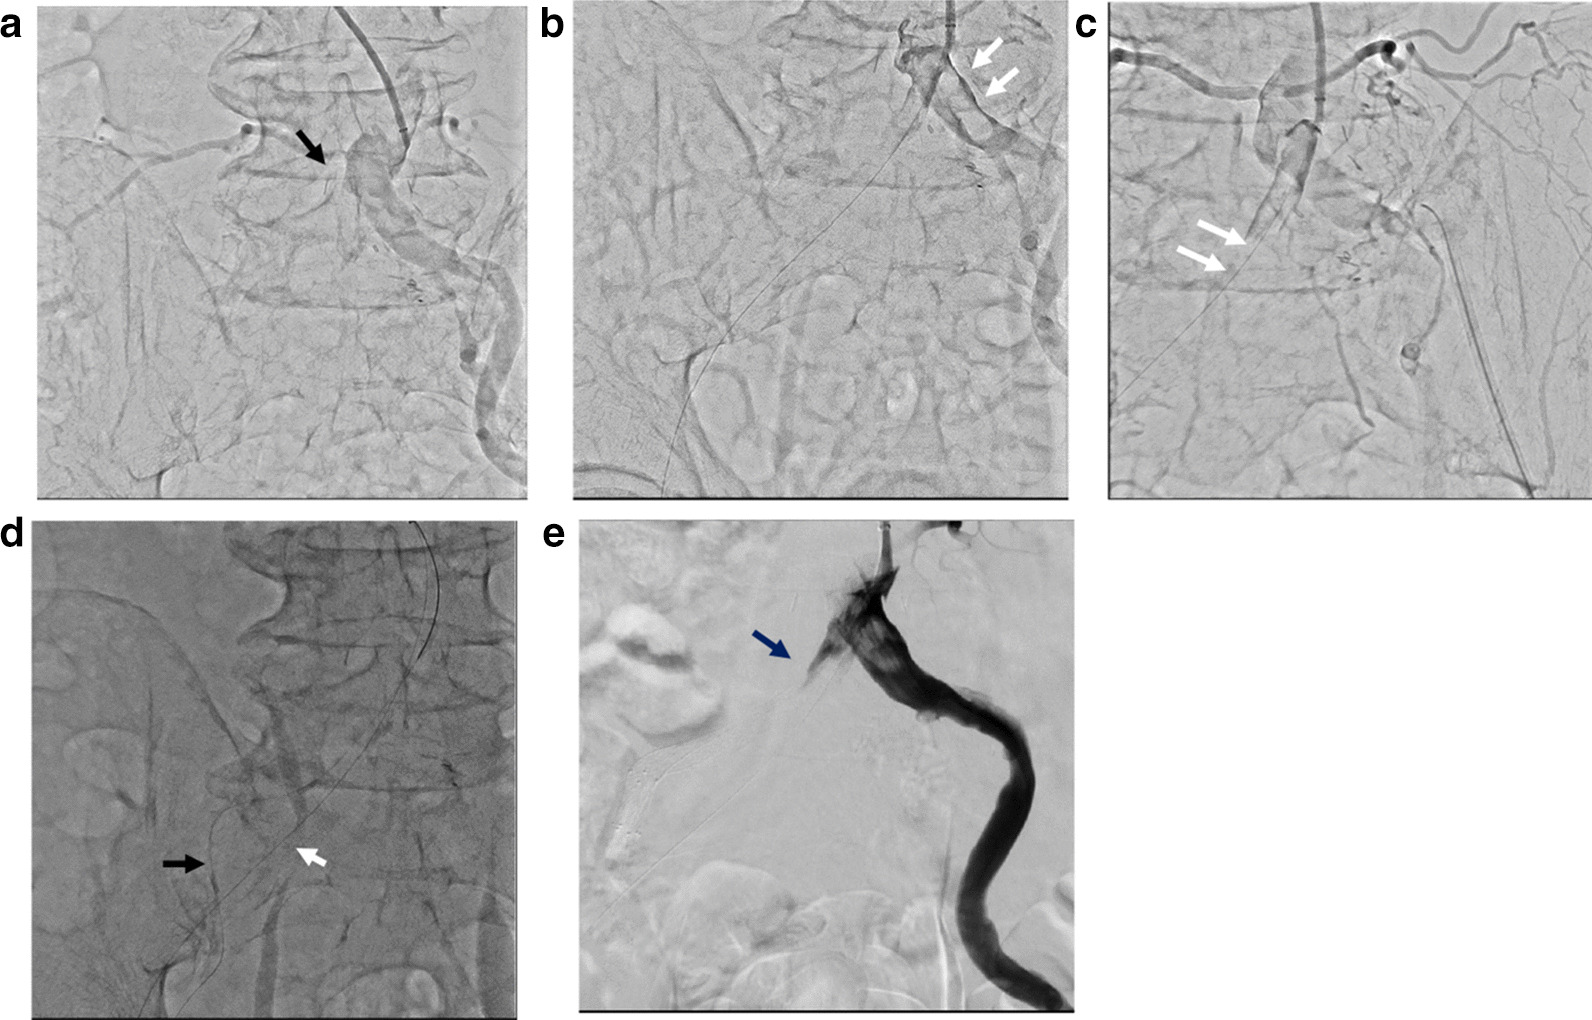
First endovascular therapy at the second hospitalization. a The native common iliac artery (CIA) and the right aortofemoral bypass were occluded (black arrow). b After ballooning the failed prosthetic aortofemoral bypass graft, the thrombus moved to the left CIA (white arrow). c The guidewire was found in the aortofemoral graft with a large amount of thrombus (white arrow). d The soft guidewire (black allow) in the native iliac artery was externalized by intravascular ultrasound guidance. A guidewire in the aortofemoral bypass graft (white arrow) was a good landmark. e Implanting two stents from the CIA to the EIA did not result in sufficient flow (black arrow)
